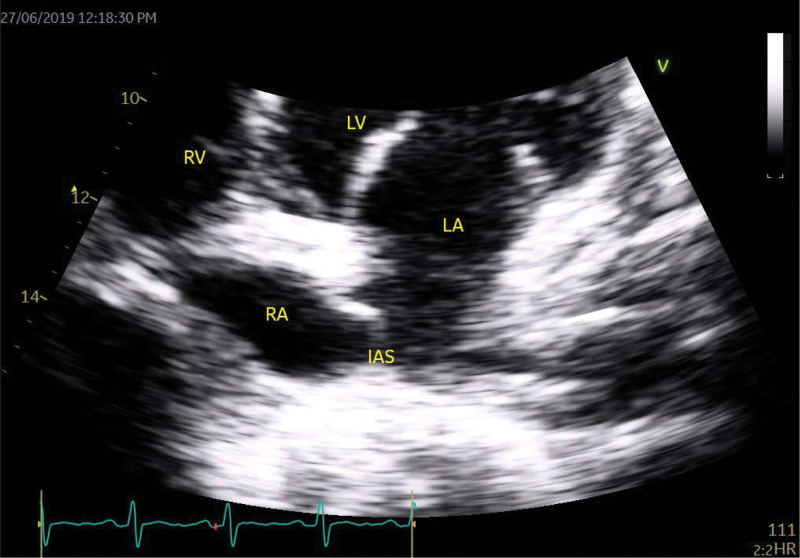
Four-chamber echocardiography view showing redundant interatrial septum frequently encountered with PFO. IAS = interatrial septum, LA = left atrium, LV = left ventricular, PFO = patent foramen oval, RA = right atrium, RV = right ventricular.
